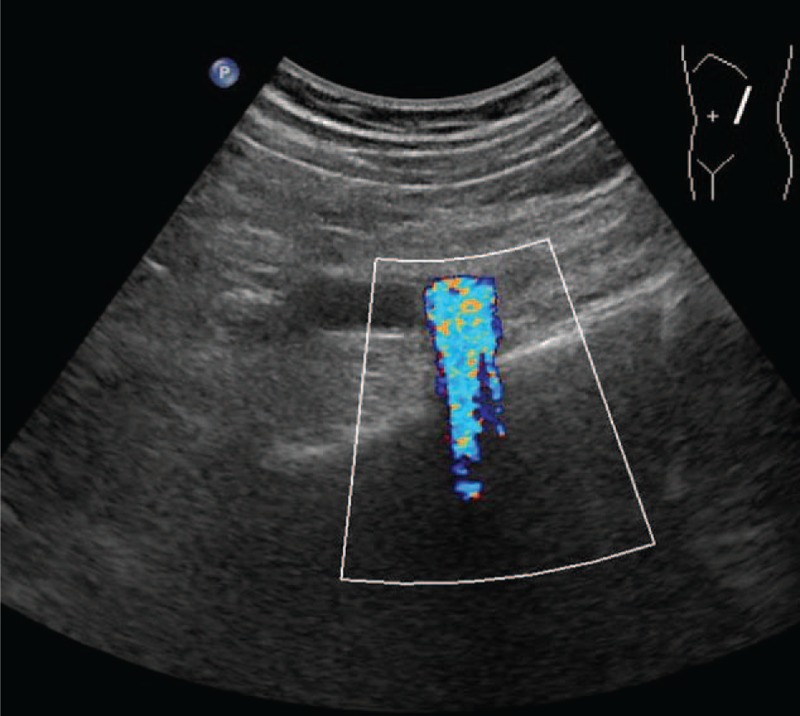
Color Doppler imaging to assess twinkling artifacts.
